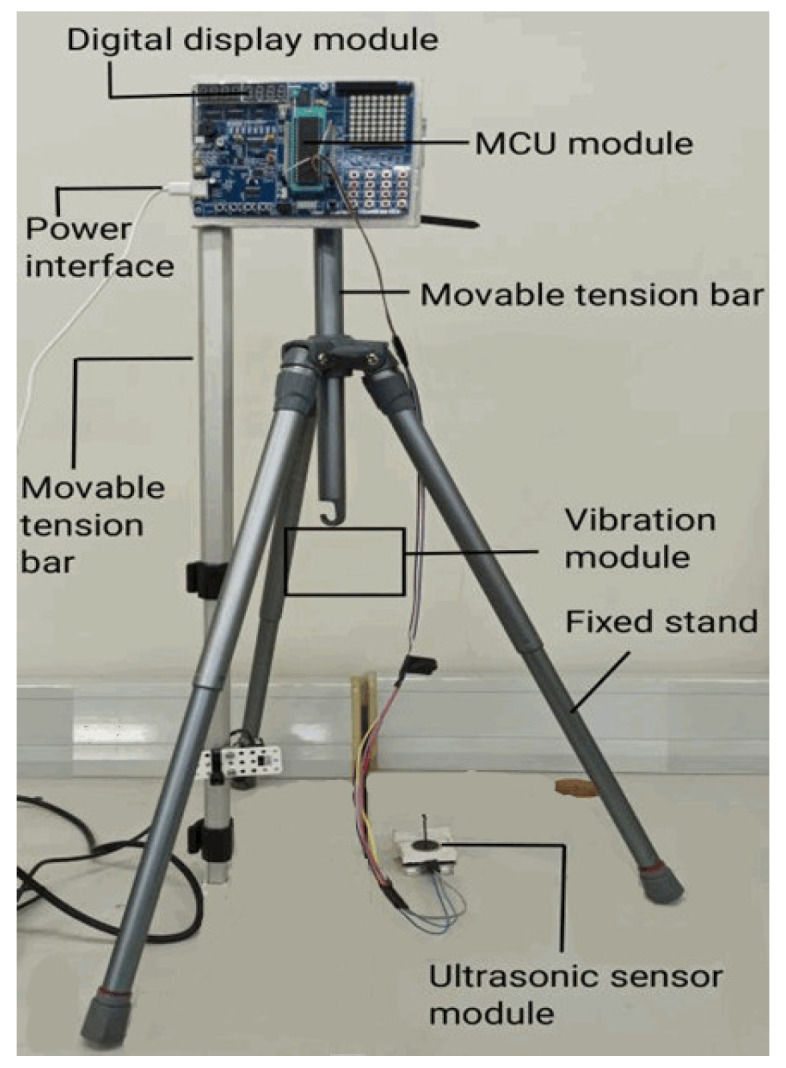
Photo of our ultrasonic testing instrument (ultrasonic sensor module was fixed together with the vibration module during use).
